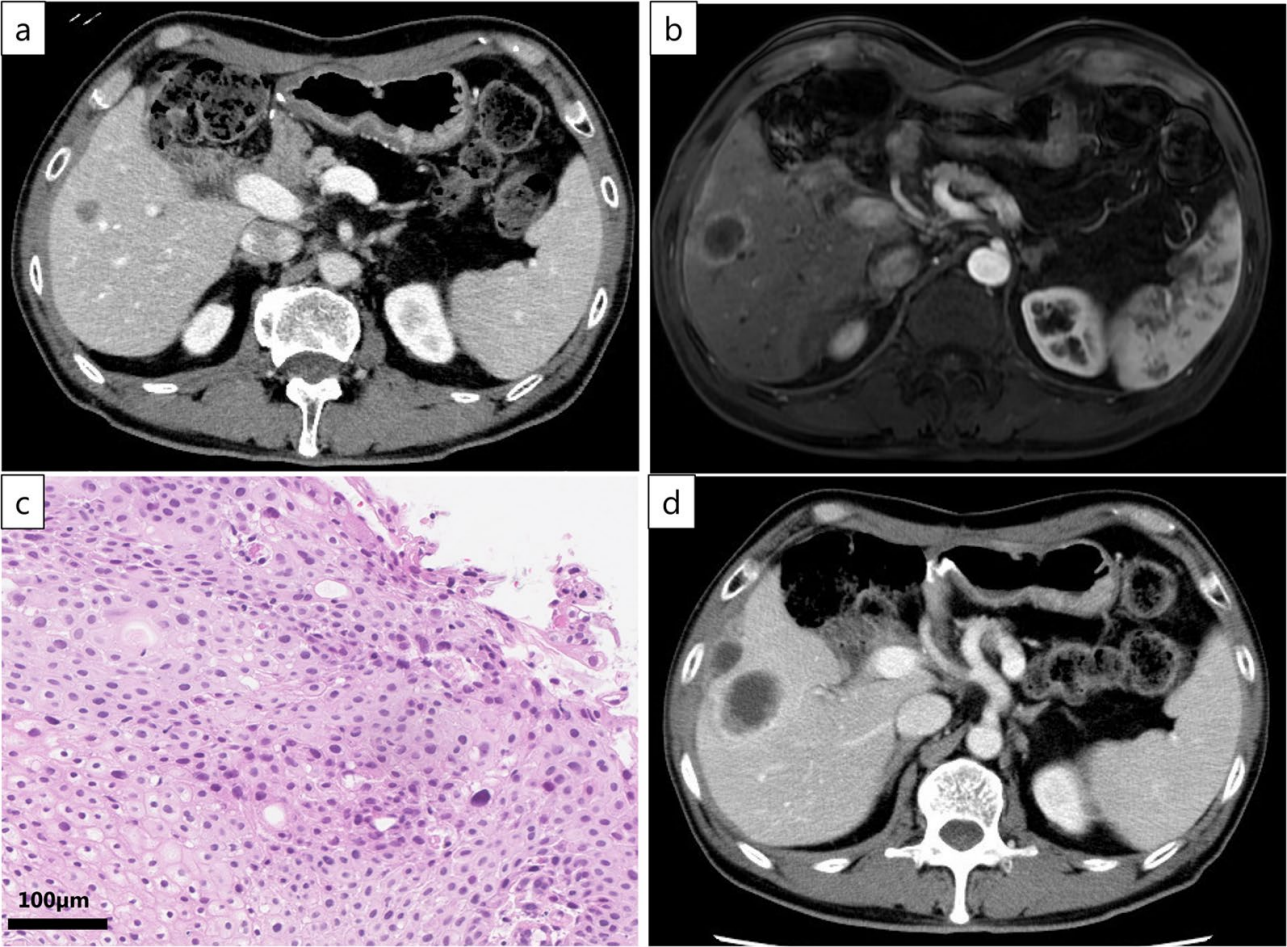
Computed tomography (CT) scan, magnetic resonance imaging (MRI) and pathological findings of the liver tumor. a Three years after pancreatectomy, CT revealed a 3-cm tumor lesion in the right lobe of the liver. b The tumor was depicted on gadoliniumethoxybenzyl diethylene triaminepentaacetic acid-MRI as enhanced peritumor in the early phase. c Atypical cells with large nuclei were growing in sheets, suggesting squamous cell carcinoma. d Three months later, CT showed that the liver tumor had grown to 6 cm in diameter
